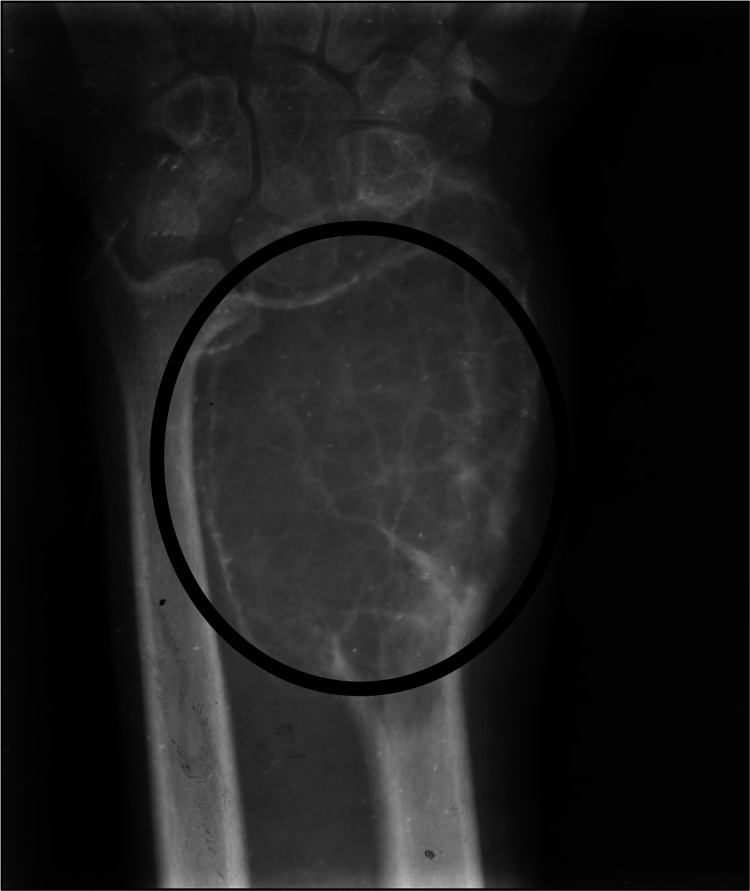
X-ray of wrist joint showing GCTB in the lower end of radius. Image taken by Yash Jha. GCTB: giant cell tumour of bone
